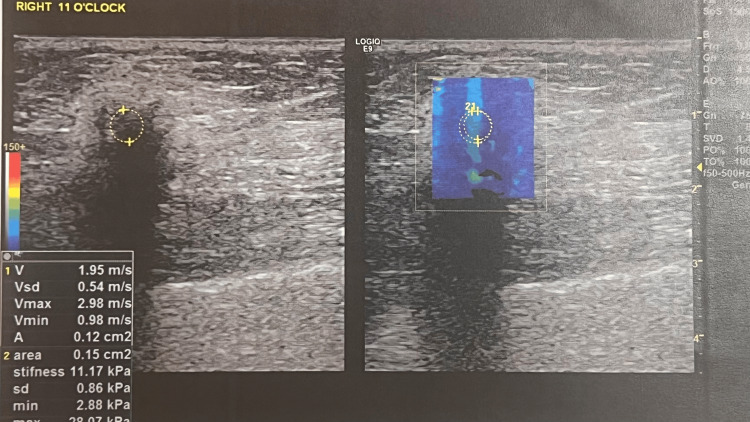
Right breast ultrasound depicting a hypoechoic lesion with posterior acoustic shadowing.
